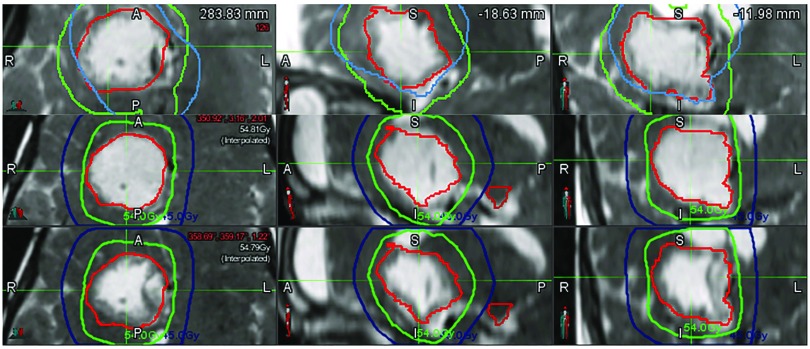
Dynamic cyst changes. Pictured are the T2-weighted magnetic resonance images (MRIs) in an axial, sagittal, and coronal presentation (left to right) taken weekly during radiotherapy for craniopharyngioma. The most superior MRI was obtained for radiation planning purposes, and the red line represents the contoured gross tumor volume. The images in the second row were obtained during the first week of radiation treatment and demonstrate cyst growth. The most inferior images were obtained during the second week of radiation treatment after the cyst had been drained via the Ommaya reservoir. The figures are original images taken in our clinic for this publication.
